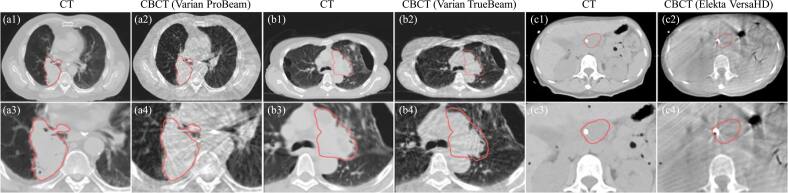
Comparisons of image quality between CT and CBCT acquired from Varian and Elekta systems. (a1)–(a2) show images from CT and CBCT on the Varian ProBeam system (125 kVp), (b1)–(b2) show images from CT and CBCT on the Varian TrueBeam system (125 kVp), and (c1)–(c2) show images from CT and CBCT on the Elekta VersaHD system (120 kVp). (a3)–(a4), (b3)–(b4), and (c3)–(c4) present zoomed-in views of regions near the tumor volume, highlighted with red contours in (a1)–(a2), (b1)–(b2), and (c1)–(c2). All figures were prepared de novo for this review using institutional data.
